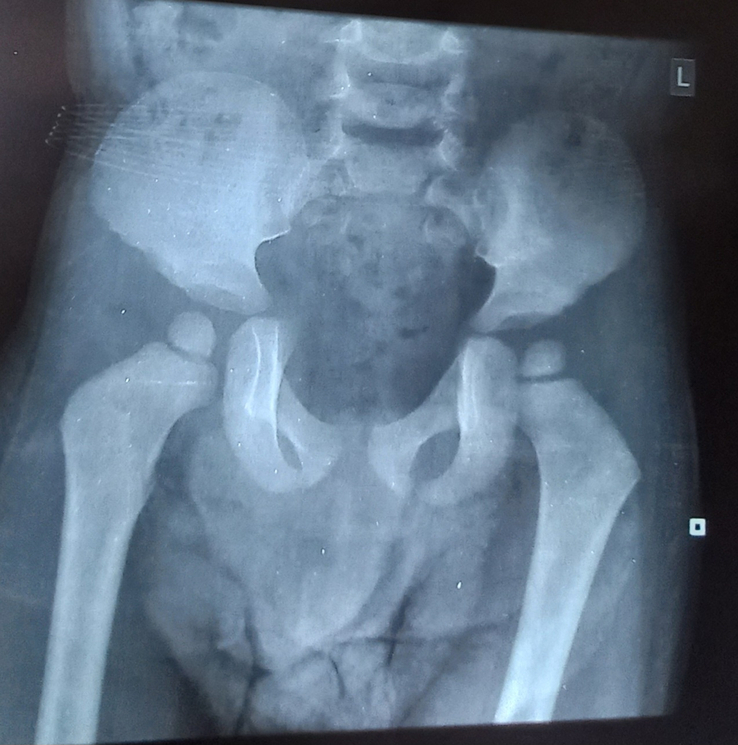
X-ray pelvis with bilateral hip joints showing mild rounding of hip bones bilaterally.
